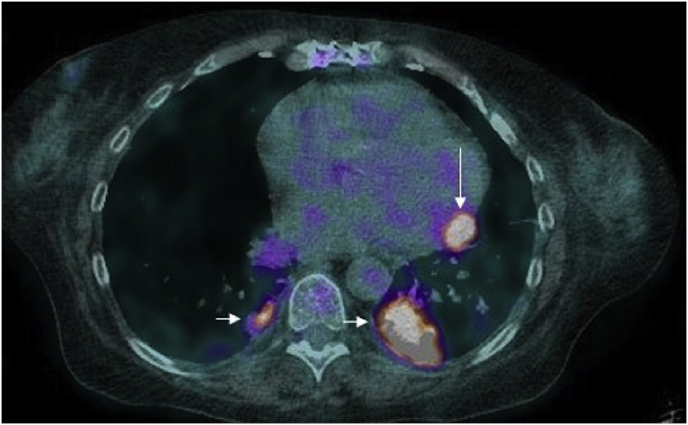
TEP image fusion highlighting hypermetabolic pulmonary lesions (horizontal arrows) and pericardial lesion (vertical arrow). Fig. 1C, Fig. 1DC and D: Left: Brain MRI frontal section showing the multi-loculated right frontal lesion with important vasogenic edema. Right: Axial section of the same lesion in diffusion-weightened imaging (DWI).
